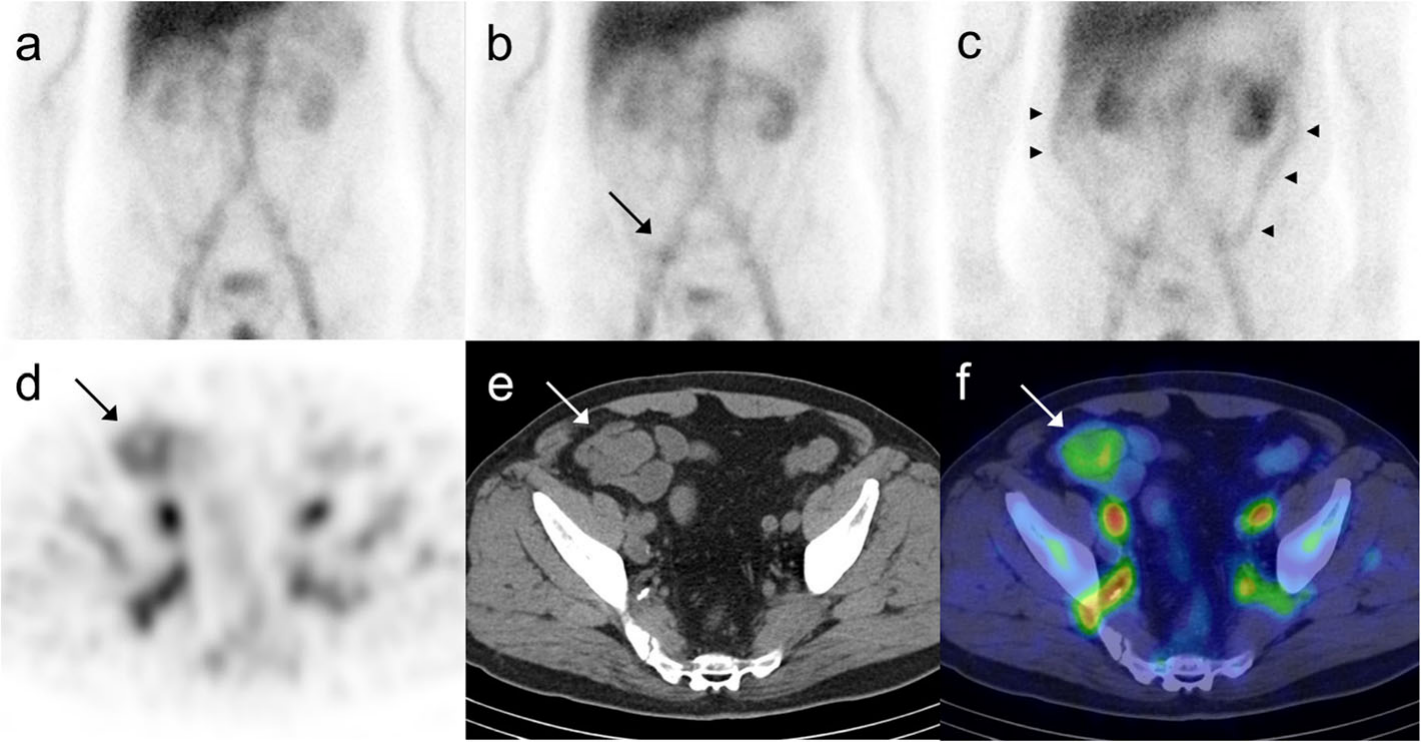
Planar + SPECT/CT of the representative patient where the site of bleeding could be detected. Planar images (a 3 h after radioisotope injection, b 6 h after, c 24 h after) and SPECT/CT images (d SPECT, e CT, f SPECT/CT, 6 h after radioisotope injection). No abnormal uptake was noted in planar images at 3 h after radioisotope injection (a). A spotty uptake was seen in the right lower abdomen in planar images at 6 h after the injection (arrow) (b). In the planar images at 24 h after the injection, a diffuse uptake was seen in the ascending and descending colon (arrowheads) (c). In SPECT/CT images at 6 h after the injection, the spotty uptake matched the distant jejunum (d, e and f) (arrows). It was revealed to be small intestinal metastasis of colon cancer
